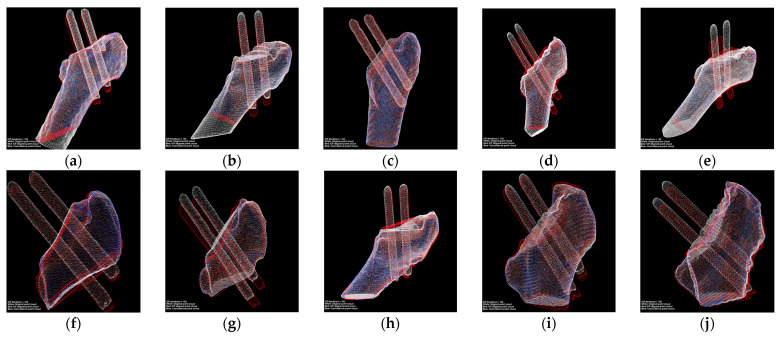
Transformation using the matrix obtained from the registration. (a–j) are the point clouds after fine registration for each of the 10 cases. The white point cloud is generated based on the postoperative CT images. The red point cloud is transformed form the point cloud generated based on the CT images scanned after one year. The blue point clouds are the coincident points after point cloud registration.
